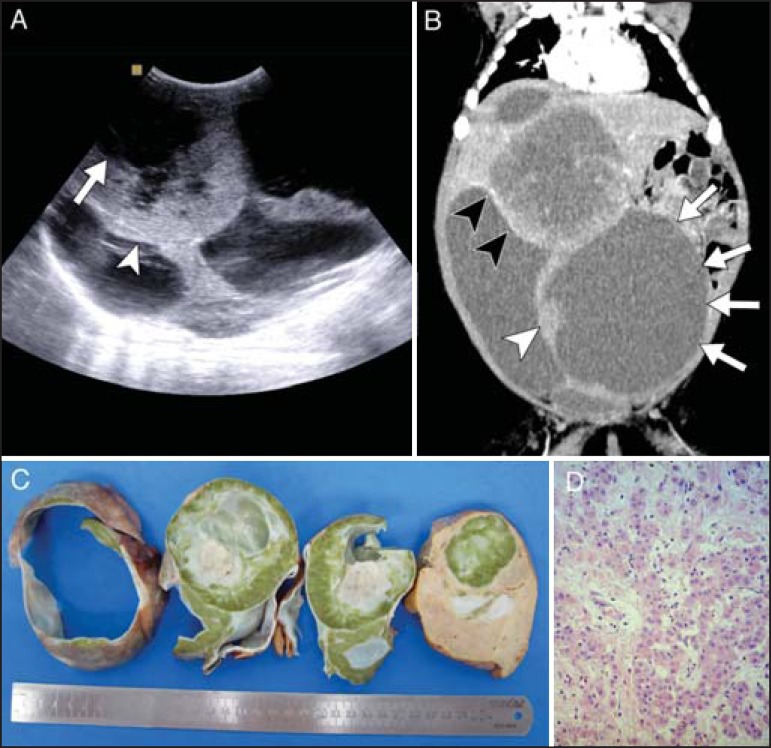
A: Ultrasound demonstrating a large heterogeneous mass
